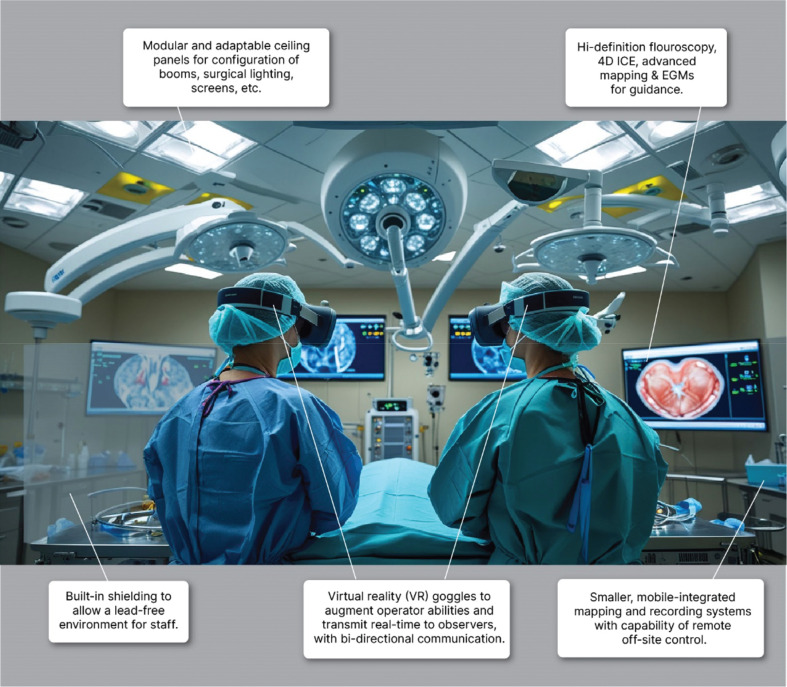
Visual display with design elements of a proposed electrophysiology lab of the future. *Abbreviations:* 4D ICE, four-dimensional intracardiac echocardiography; EGM, electrogram.
